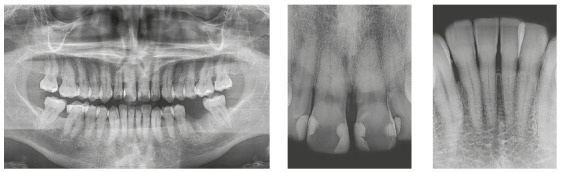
Initial radiographs of Case 4.
